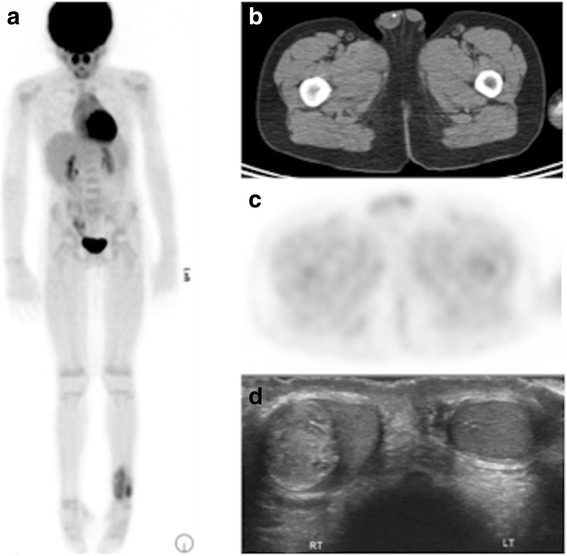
The attenuation correct CT has diagnostic value. Eight years old patient with Ewing sarcoma of the left distal tibia. ^18^F-FDG PET/CT shows uptake in the primary tumor, but no metastatic disease (a). Scrotal calcification was detected incidentally on the AC CT (b), but was not associated with FDG uptake (c). Ultrasound confirmed a mass (d), which was revealed to be a mature teratoma, unrelated to the primary tumor
